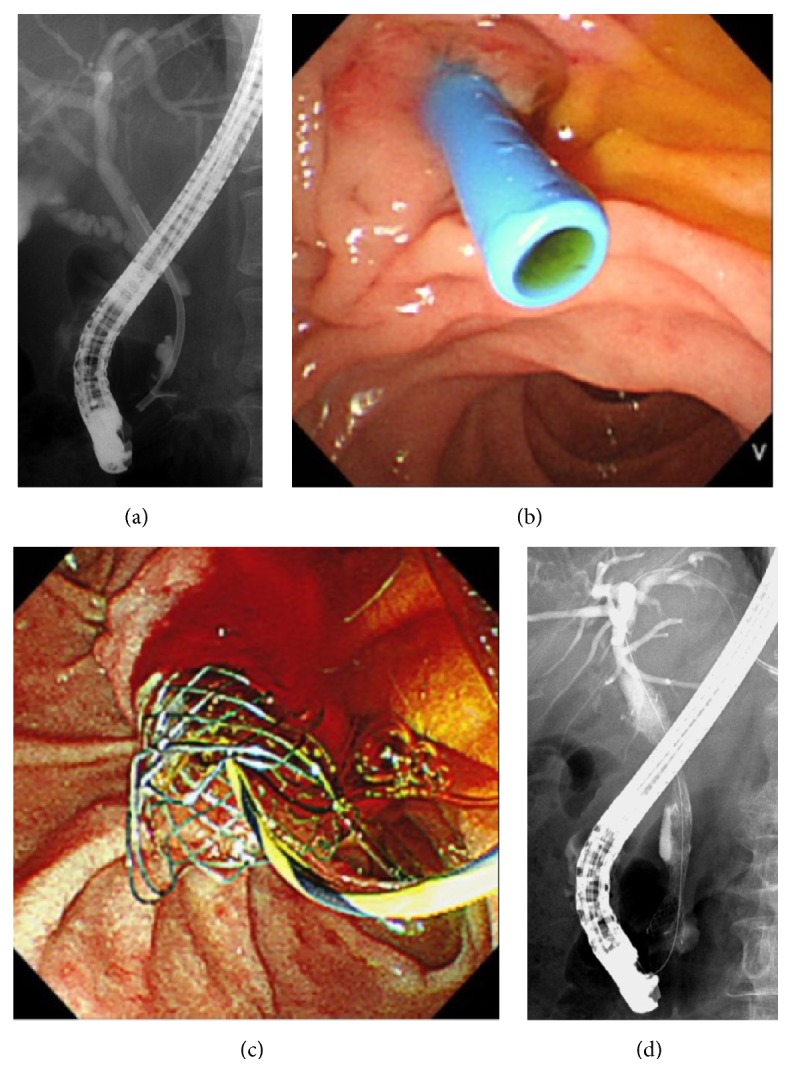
Endoscopic biliary drainage. (a), (b) X-ray image and endoscopic image of plastic stent. (c), (d) X-ray image and endoscopic image of metallic stent.
